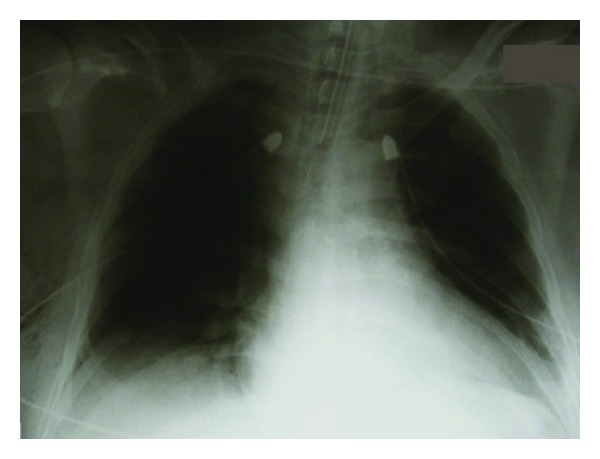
Portable chest radiograph showing a right-sided haemothorax and a widened mediastinum (bilateral chest drains inserted).
